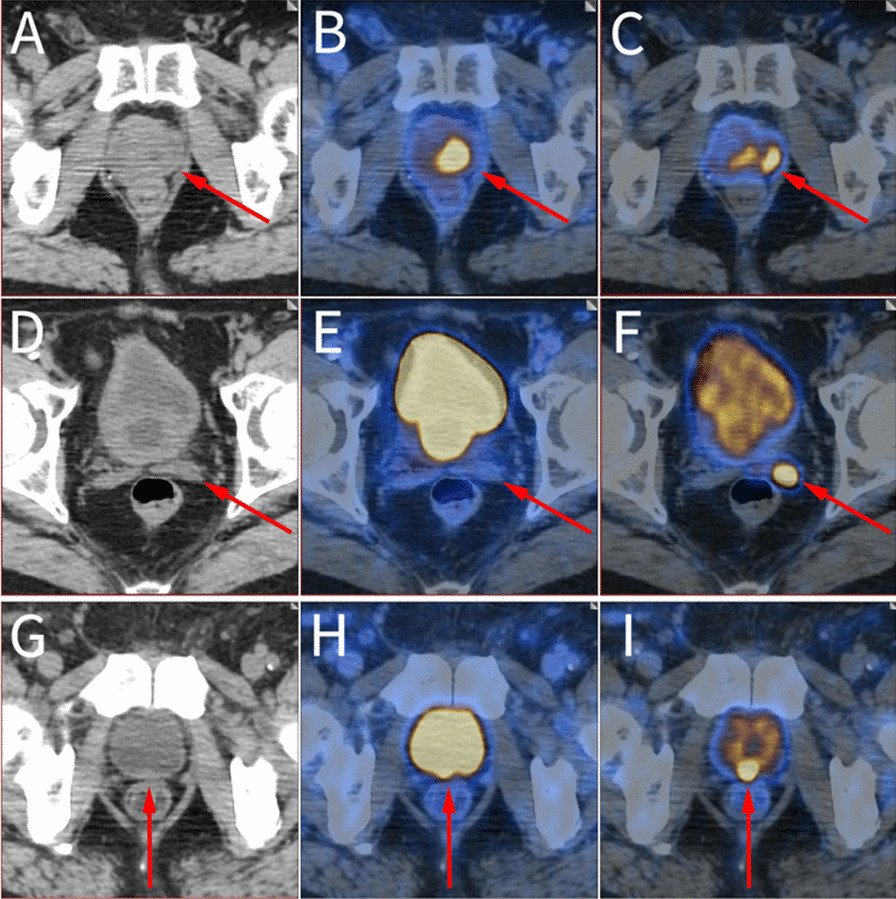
Delayed images showed additional lesion detection in prostate region. [^68^ Ga]Ga-PSMA LFOV PET/CT in a 78-year-old primary PCa patient after TURP (tiles A–F). The high radioactivity within the bladder and enlarged prostatic urethra impeded the detection of PSMA-avid lesions in early images (tiles B and E). A locally lesion at the left lobe of prostate gland was discernible in delayed image, with a SUV_max_ value of 9.6 (tile C). In addition, late scan detected invasion of left seminal vesicle (tile F), which was not distinguishable in the early scan, the SUV_max_ value was 1.7 in the early image, while the delayed image showed a SUV_max_ value up to 15.0. [^68^ Ga]Ga-PSMA LFOV PET/CT in a 70-year-old BCR patient (tiles G–I). A locally recurrent lesion at the prostate bed was detected at the delayed images, with a SUV_max_ value of 25.8 (tile I), which was unclear in early image due to the high radioactivity within the bladder (tile H)
